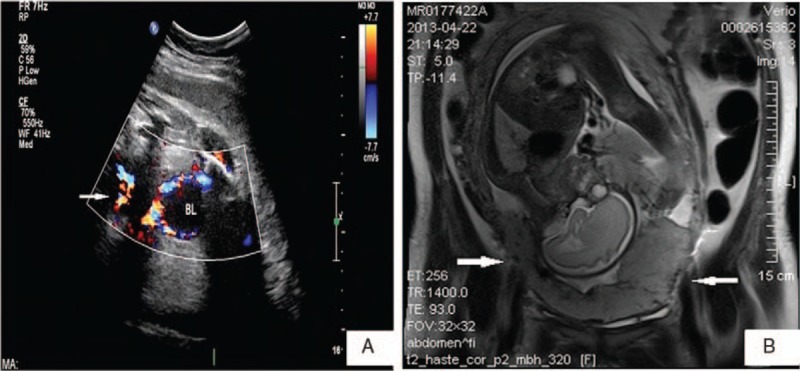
A. Ultrasonagraphy showing multiple hypoechoic spaces on the placenta (denoted by arrows). B. Axial T1-weighted MRI image showing complete placenta previa with invasion into the anterior/posterior myometrium of the uterus, suggestive of placenta increta (denoted by arrow).
